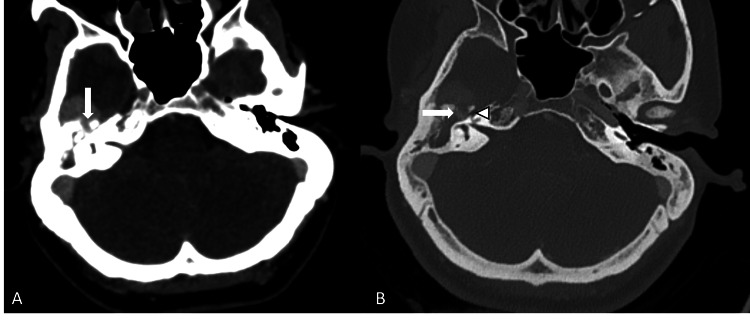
(A) Axial CECT image demonstrating a tongue-like projection of the enhancing tumor into the right petrous bone (arrow). (B) Axial HRCT image demonstrating dilated hiatus for the greater petrosal nerve (arrow). The genu of the facial nerve is marked by an arrowhead. CECT: contrast-enhanced CT; HRCT: high-resolution CT
